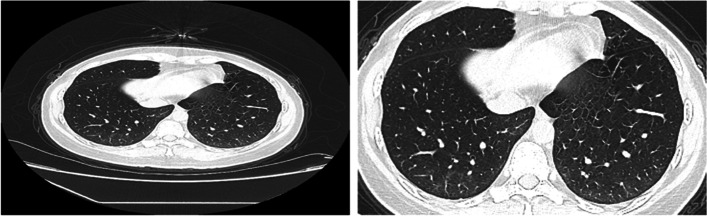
Sample chest CT image from CoDS2 before (left) and after (right) cropping
